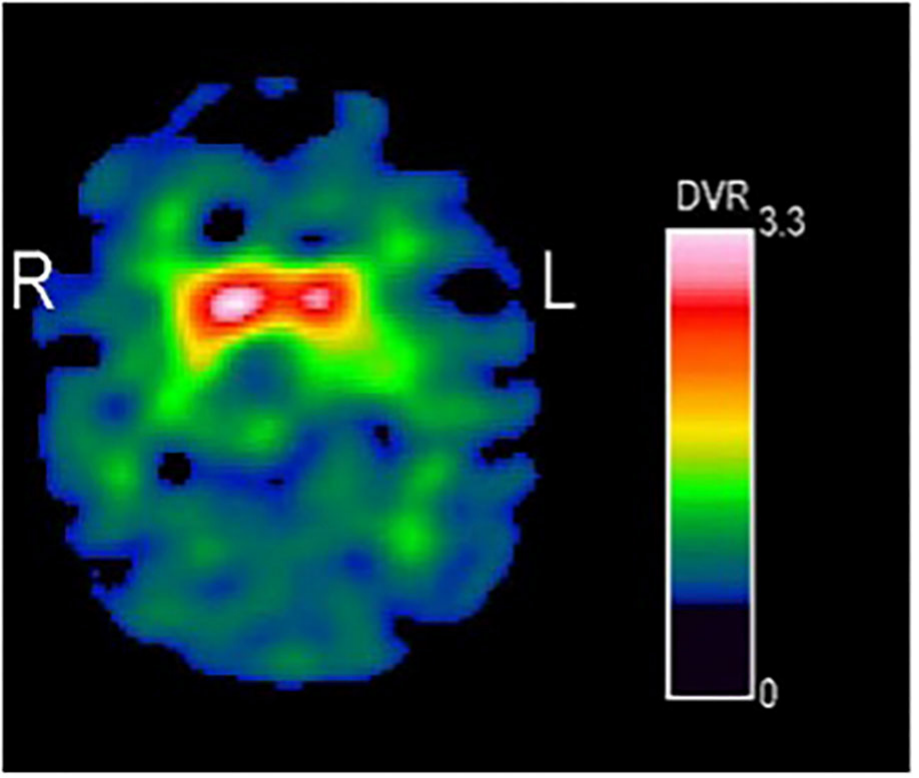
[^123^I] FP-CIT-SPECT of our EOPD patient showed profoundly reduced binding at dopamine transporters on nigrostriatal neurons. Reductions were slightly more pronounced on the left side for the caudate nucleus (right: −41%; left: −48%) and the putamen (right: −67%; left: −71%) as compared to the age-related reference (DaTQUANT^TM^ software, GE Healthcare).
